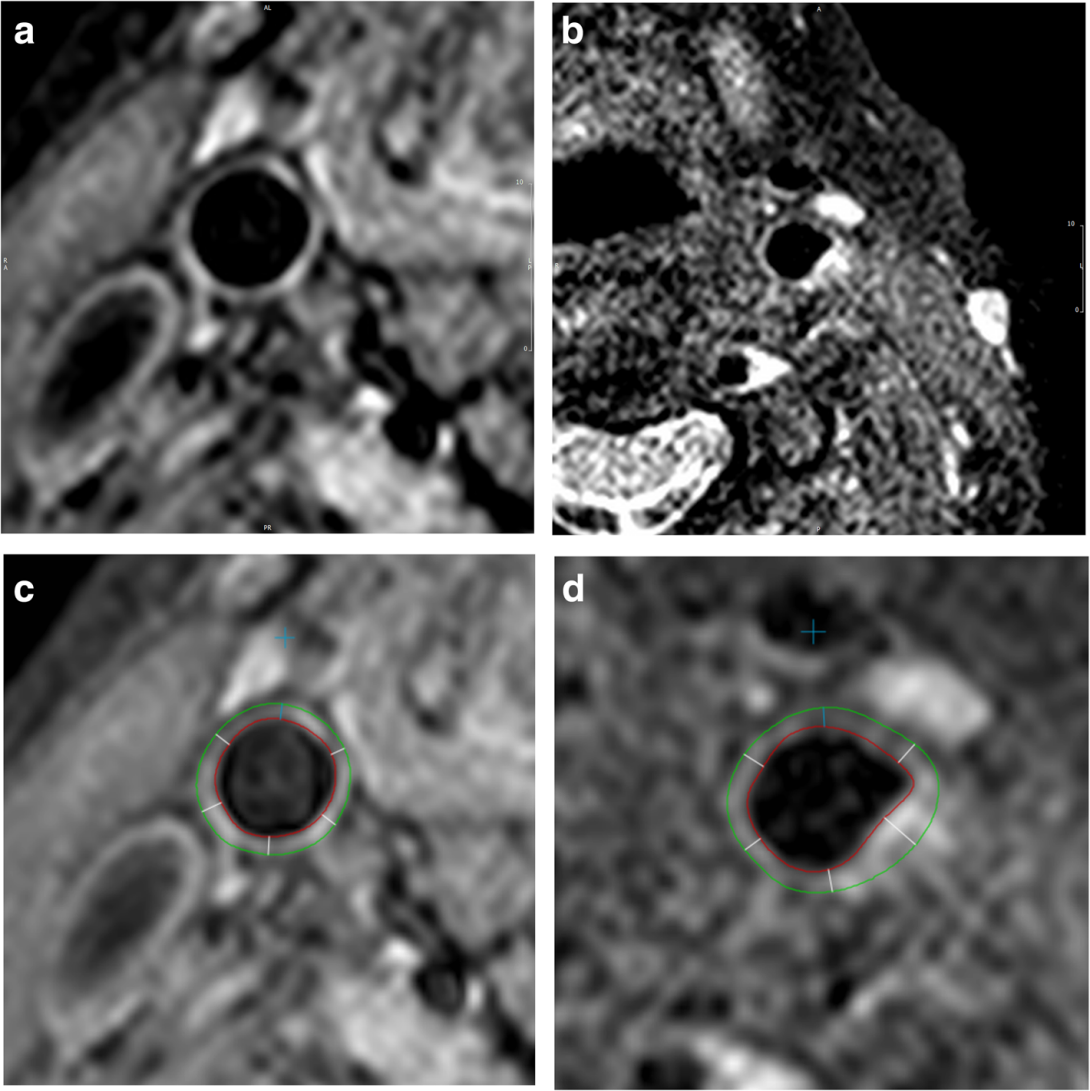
Cross-sectional T1-weighted images of the carotid artery in short-axis views. a Normal carotid artery, b carotid artery with plaque, c normal carotid artery with contours, d carotid artery with plaque with contours. Red lines represent the endothelial wall and green lines represent the outer (adventitial) wall of the carotid artery
